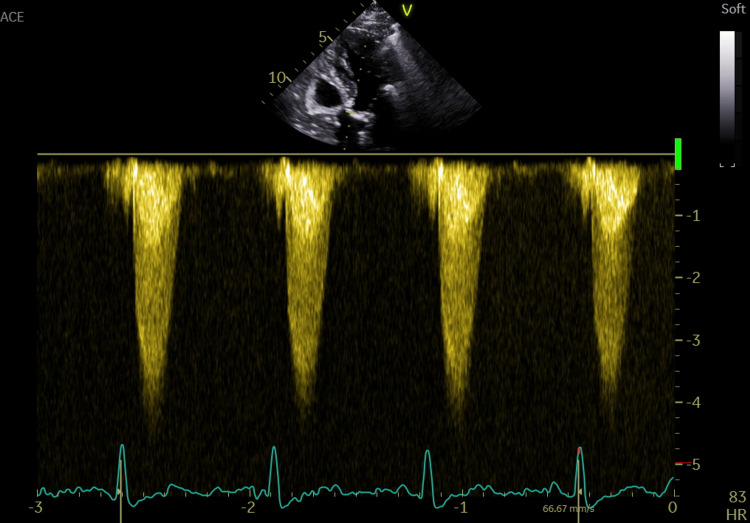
Apical 5-chamber view CW Doppler tracings showing a Peak velocity ≥ 4 m/s
